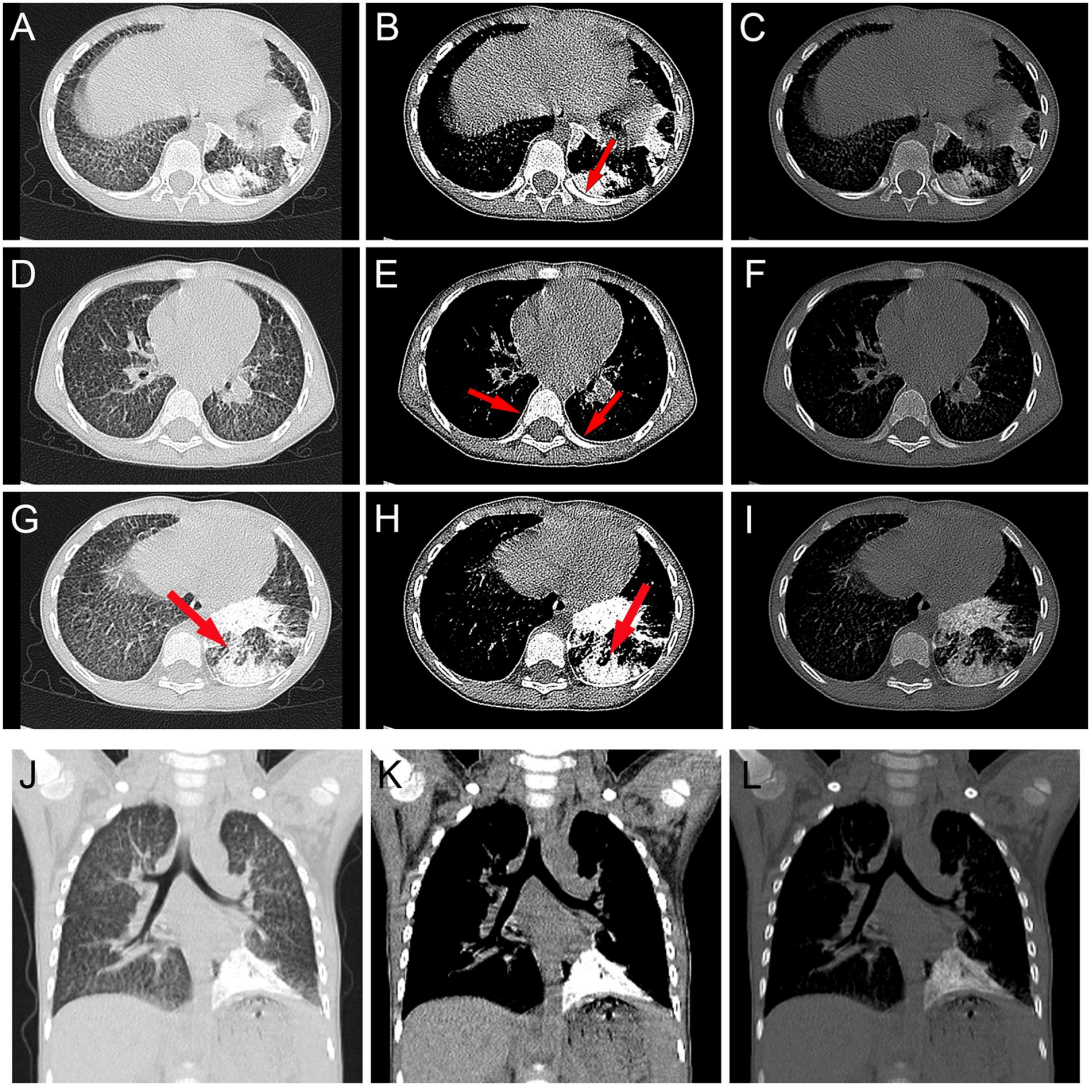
The HRCT of the patient demonstrates: (A,D,G,J) lung window, (B,E,H,K) mediastinal window, and (C,F,I,L) bone window views. Calcifications along the cardiac border and pleura were distinctly visualized in both mediastinal and bone windows. (B) Subpleural cysts between the ribs and calcified parenchyma were referred to as the “black pleura” sign (red arrow). (E) The “white line sign” (red arrow) was characterized by multiple punctate and linear calcifications. (G,H) The “flame-shaped sign” (J,K,L) Extensive calcifications are observed along the left lower pulmonary-diaphragmatic interface.
